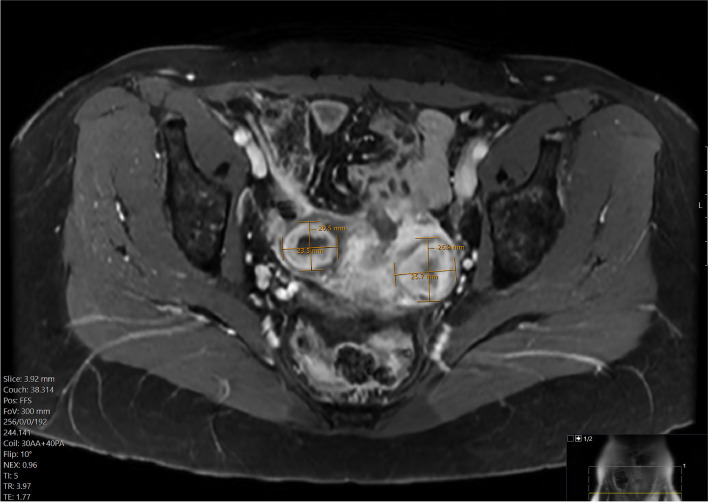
The patient’s MRI four months after embolization showed reduction in both fibroids – 23.5 × 20.5 mm on the right and 25.7 × 26.9 mm on the left
